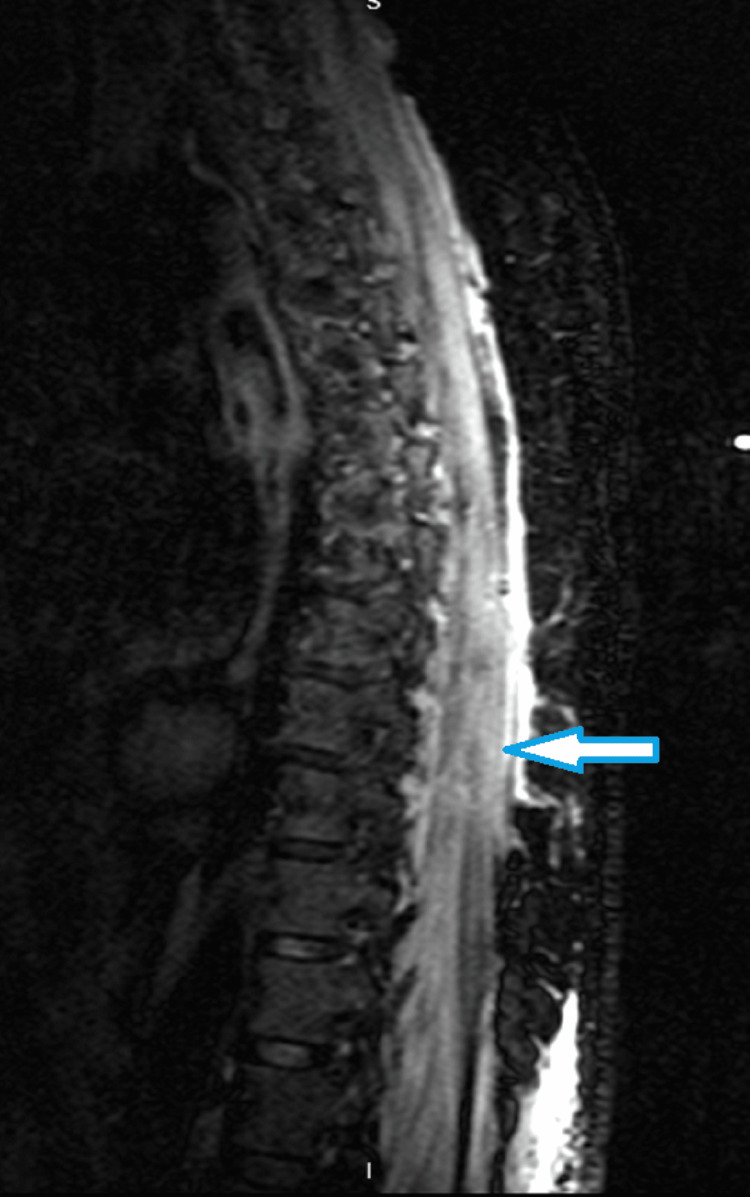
MRI of the thoracic spine, sagittal view Diffuse T2/STIR hyperdensities seen in paravertebral and thoracic musculature (arrow shows a part of the hyperdensity)
